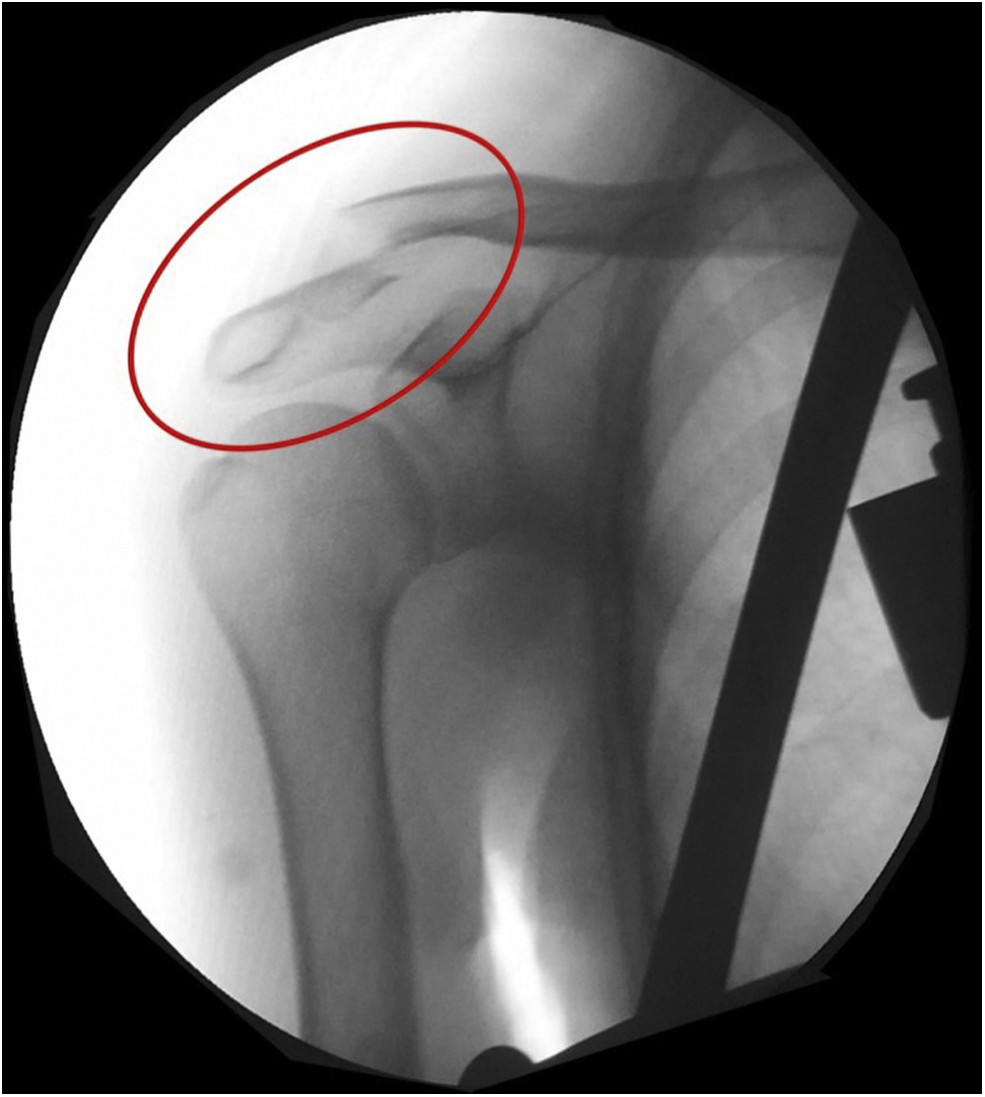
Preoperative radiographic fluoroscopic image of the shoulder (anteroposterior view). Acromioclavicular fracture-dislocation.
